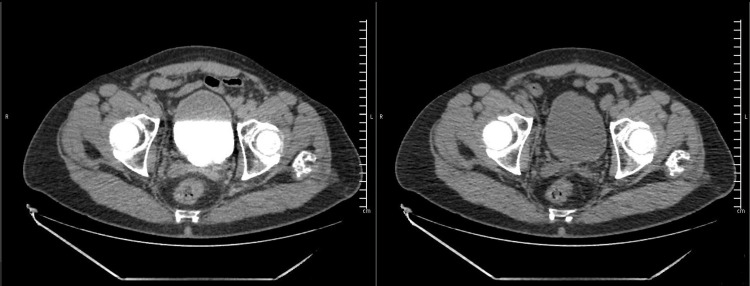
Pelvic CT scan confirming the complete resolution of the pelvic hematoma.
